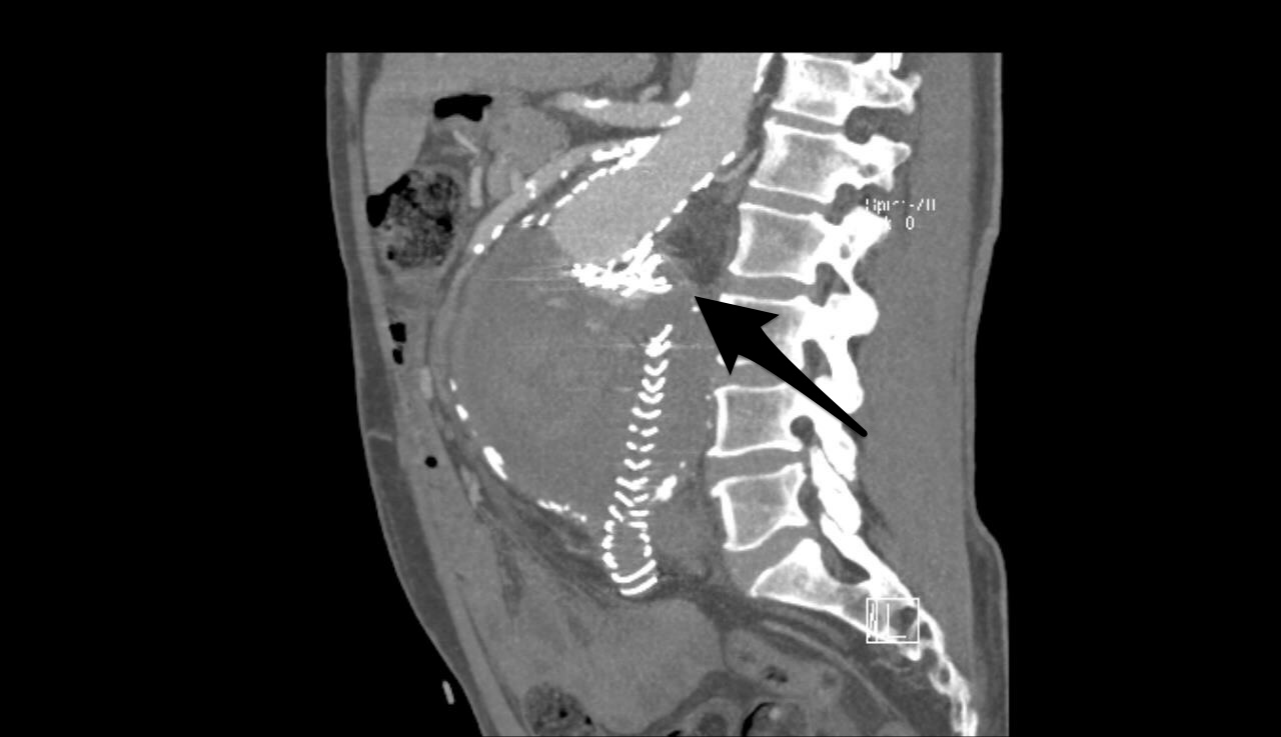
Post-procedure 62-month CT-scan depicting a large, type III endoleak (graft disconnection arrowed) and enlargement of the aneurysm to 133 mm in diameter.
